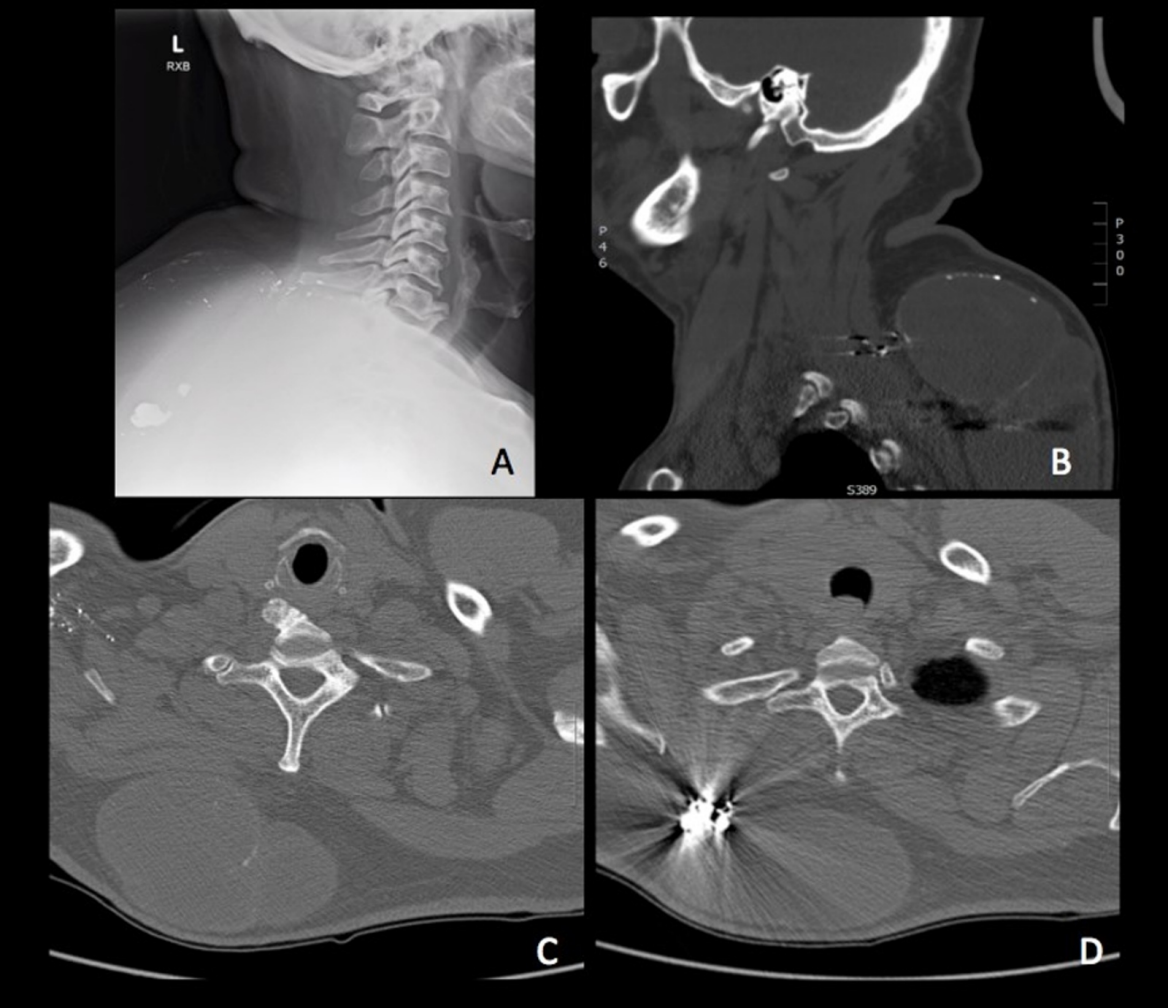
Well-circumscribed, multilobulated subcutaneous lesion with thin septation and ballistic fragments along the margin. Cervical x-ray (A); Non-contrast CT: Sagittal view (B), Transverse views (C and D)
